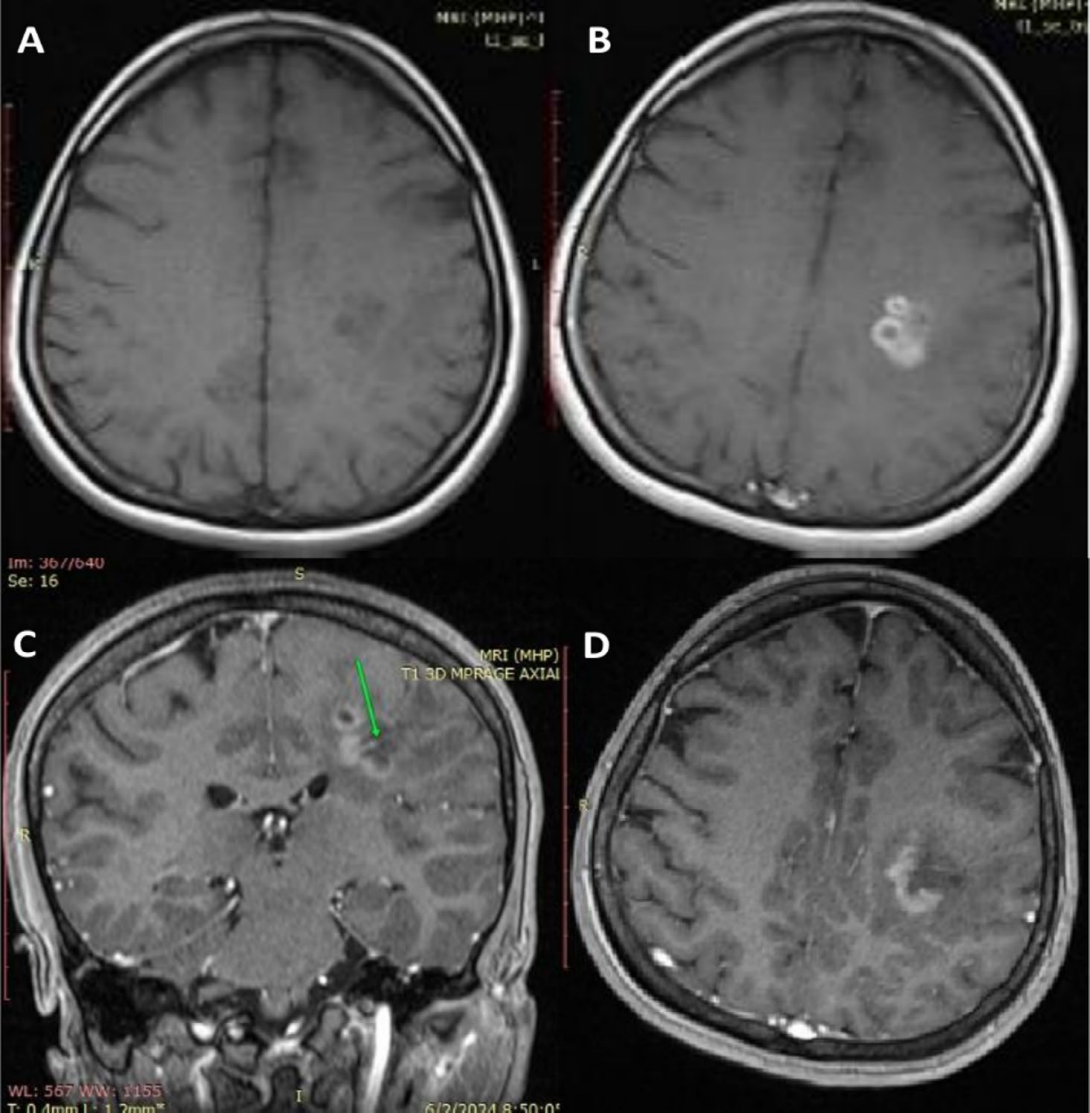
Contrast enhanced sequences of first brain MRI 2 months after initial presentation. (A) T1 Axial Pre Contrast, (B) T1 Axial Post Contrast, (C) T1 Coronal Post Contrast, (D) T1 Axial Post Contrast. At post contrast scan, both closed ring and open ring type of enhancement seen with open side of the ring facing gray matter, green arrow indicating there is an enhancing prominent medullary vein.
